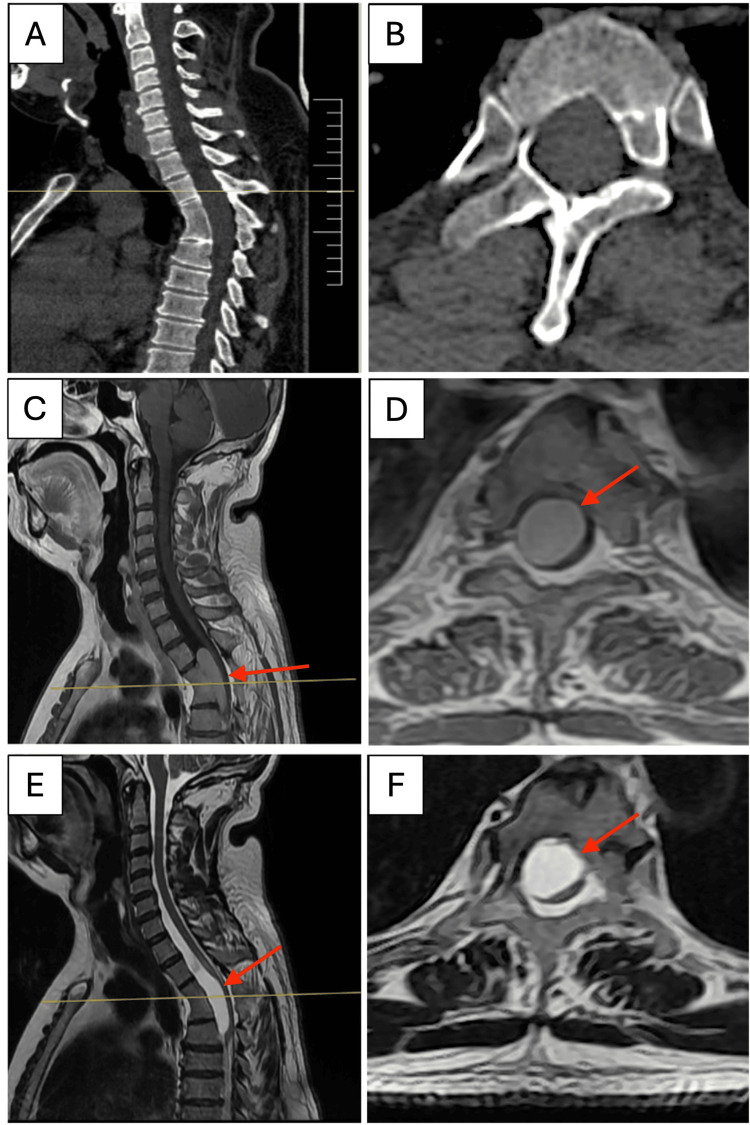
Preoperative imaging (A)-(F) Magnetic resonance imaging (MRI) and computed tomography (CT) scan for diagnostic assessment. (A)-(B) Sagittal and axial CT scan sections show the vertebral fusion at T3-T4 levels, with thoracic kyphosis increased and a widened spinal canal. (C)-(D) MRI at T1 and (E)-(F) T2 sequences with sagittal and axial sections. MRI displayed the fusion of the vertebral bodies at the T3-T4 levels, with a hyperintense lesion within the spinal canal (red arrows). The latter extends from the lower portion of T2-T5 levels, supporting the intradural and extramedullary elements. It also demonstrated the posterior displacement of the spinal cord and an extended area of more than 90% of the spinal canal.
